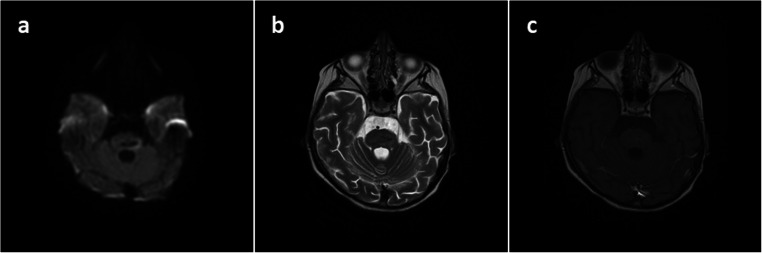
Axial cranial MRI a DWI, b T2-weighted, and c T1-weighted with gadolinium performed 1 month after admission showing nearly complete disappearance of the abscess
